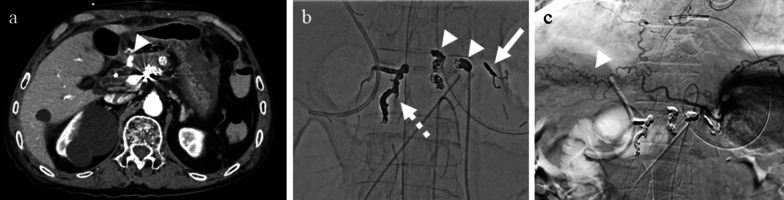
Imaging findings at postoperative day 32. a Enhanced CT showed pseudoaneurysm at the gastroduodenal artery (arrowhead). b Angiography showed the partially dislocated coil placed at the common hepatic artery (arrowhead), left gastric artery (arrow), and gastroduodenal artery (dotted arrow). c Angiography from the root of the celiac artery showed intrahepatic arterial flow (arrowhead) from the right inferior phrenic artery
